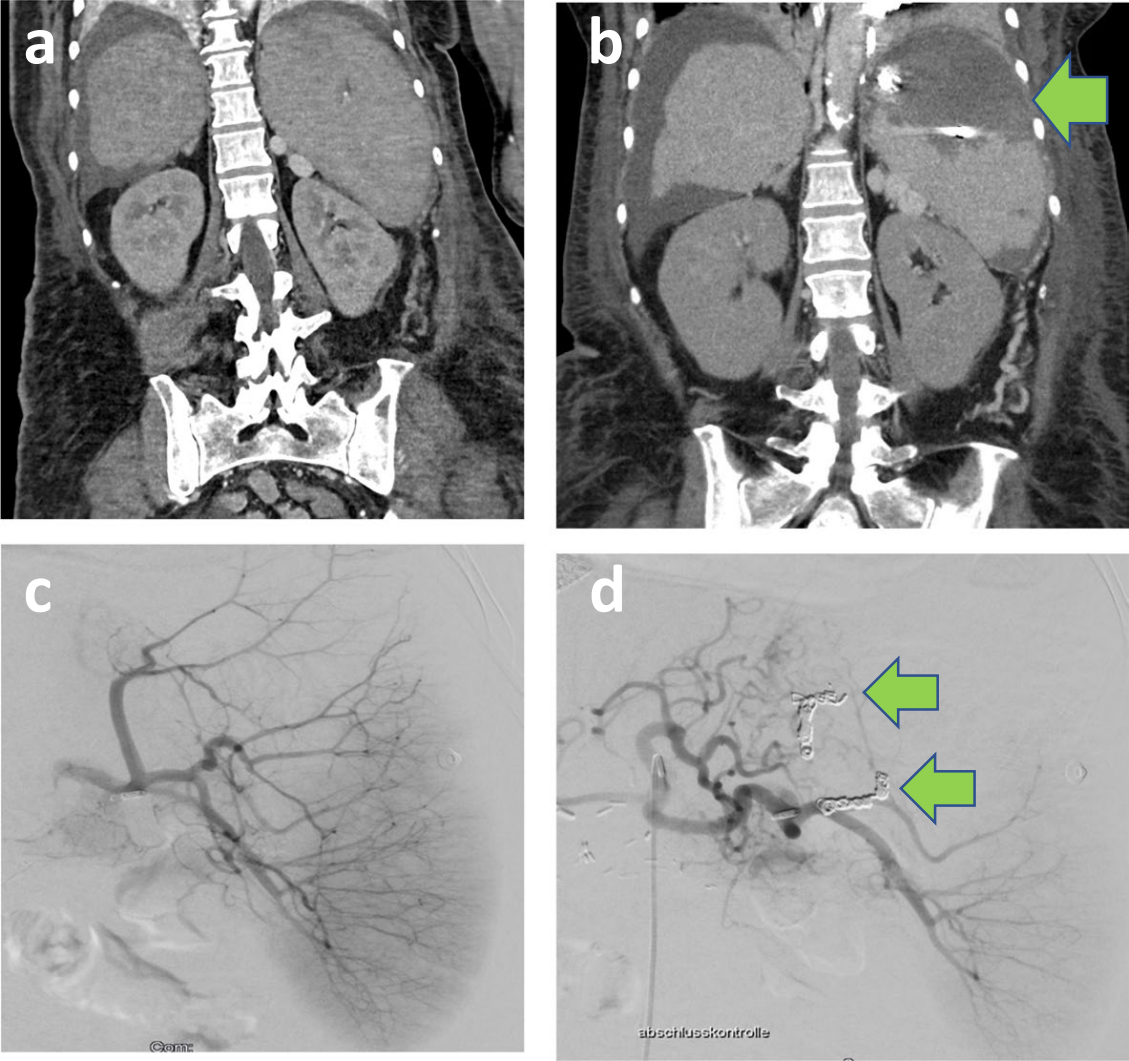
Computed tomography of a patient before (a) and after (b) partial splenic artery embolization. Angiography before (c) and after (d) partial splenic artery embolization. Embolization of the upper branches of the splenic arteries using microcoils and gelatin (marked with arrows); embolization of 60% of the splenic parenchyma d (marked with arrow)
